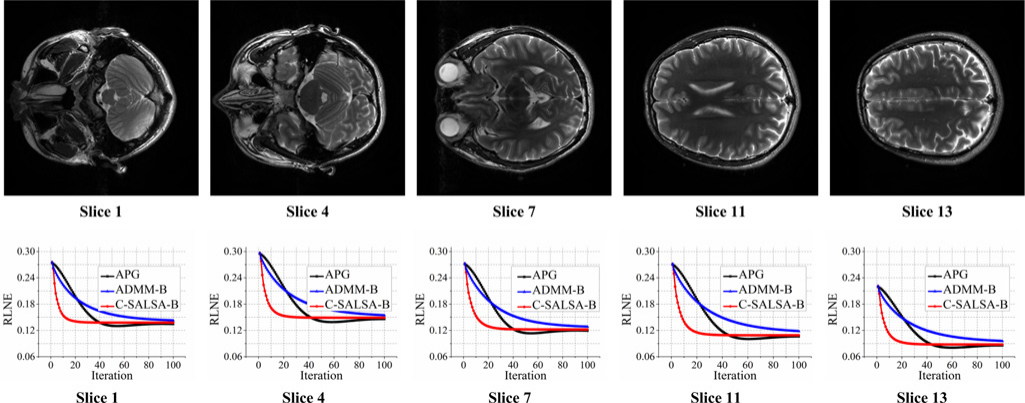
Comparisons of C-SALSA-B to APG and ADMM-B for more MR images.
